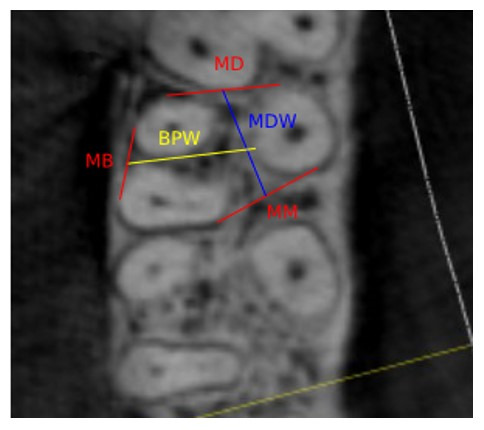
The distance (FSD) from the sinus floor (SF) to the molar furcation in the mid-most CBCT slice was recorded as described by Choi et al (Figure 3).^
